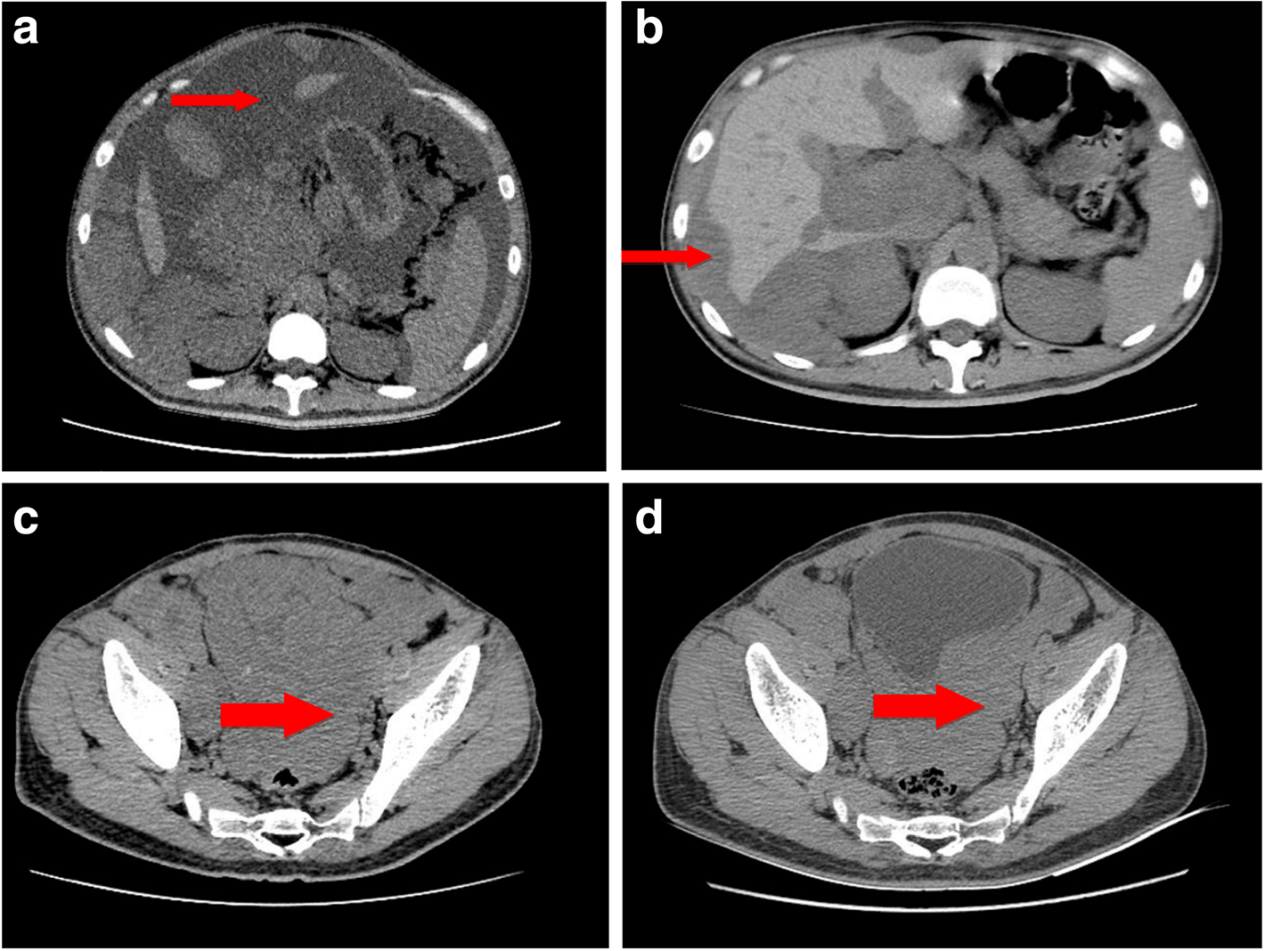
Abdominal CT scans before and after apatinib therapy. a. Before apatinib therapy (May 19, 2017), CT scan showed extensive ascites. b. After 1 month of apatinib treatment (July 3,2017), ascites decreased significantly. c. After 1 month of apatinib treatment (July 3,2017), CT scan showed the mass in front of rectum. d. After 2 months of apatinib treatment (August 7, 2017), the mass was smaller than 1 month ago(reduced in size by 40%).The thin and thick arrowheads aim at ascites and metastatic mass in front of rectum, respectively
